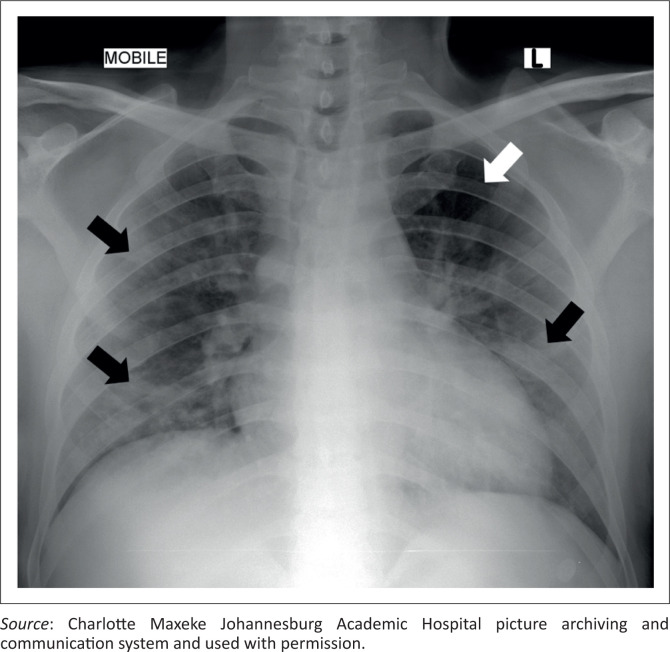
Chest radiograph of a 52-year-old man with hypertension, diabetes mellitus and chronic kidney disease who presented with fatigue and malaise. There are patchy, peripheral and central ground glass opacities bilaterally (black arrows), with sparing of the left upper zone (white arrow).
